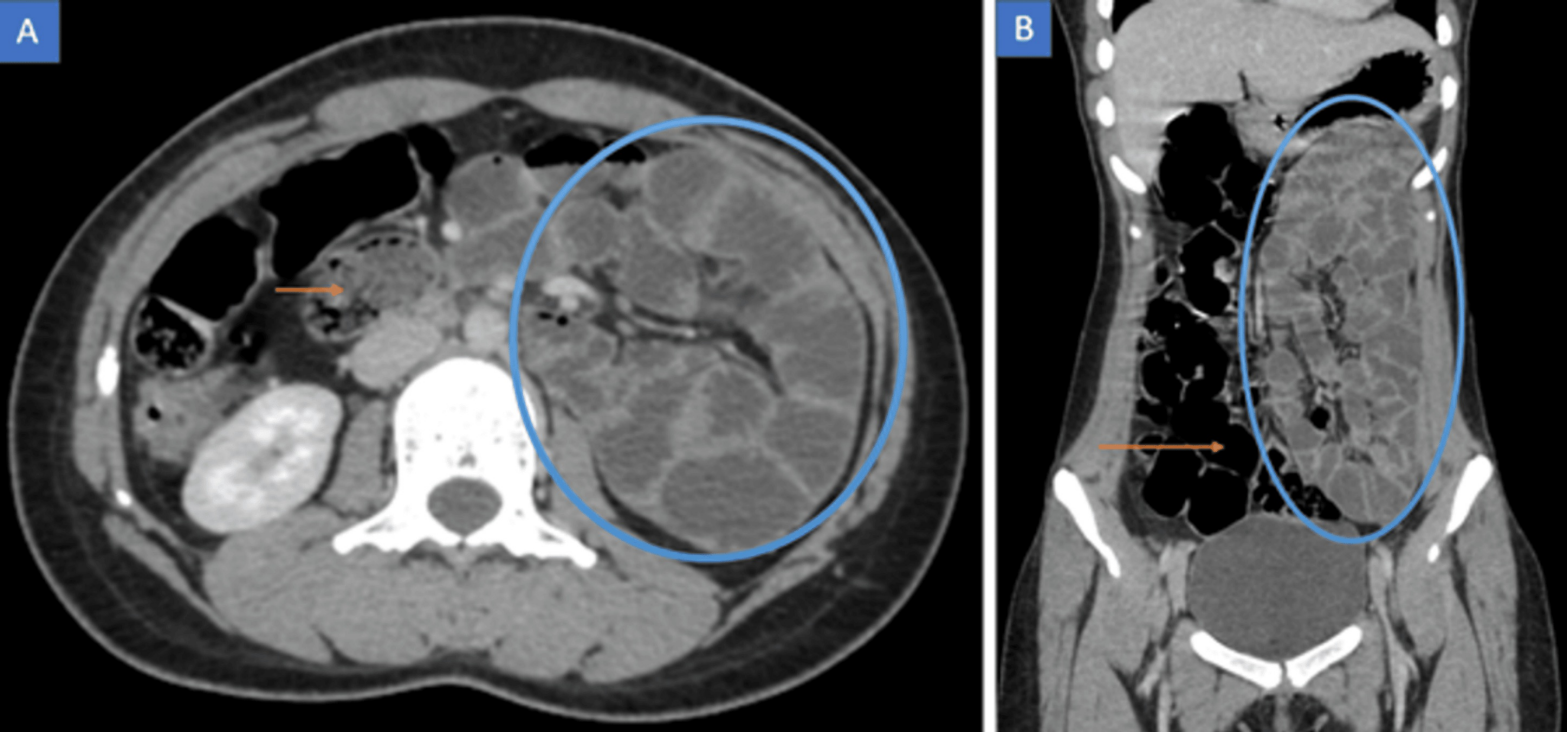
Contrast-enhanced axial and coronal CT images (porto-venous phase) (A) Contrast-enhanced axial CT abdomen image demonstrating medial displacement of the descending colon (orange arrow) with small bowel loops (blue circle) lateral to the colon occupying the left paracolic gutter. (B) Contrast-enhanced coronal CT abdomen image showing an oblique course and medialization of the descending colon (orange arrow) and lateralization of small bowel loops (blue circle).
